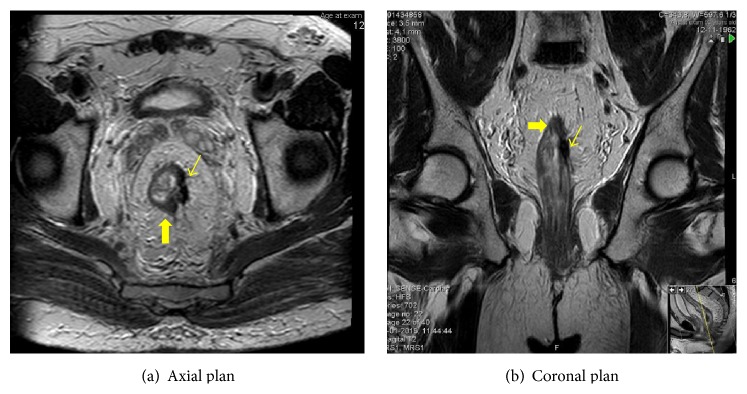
MRI 11 weeks after CRT completion. Good tumor response: tumor downsizing and downstaging, nodal downstaging, and increase between tumor and mesorectal fascia. Thin arrow: areas of fibrosis (very low signal intensity); thick arrow: areas of residual tumor (intermediate signal intensity).
